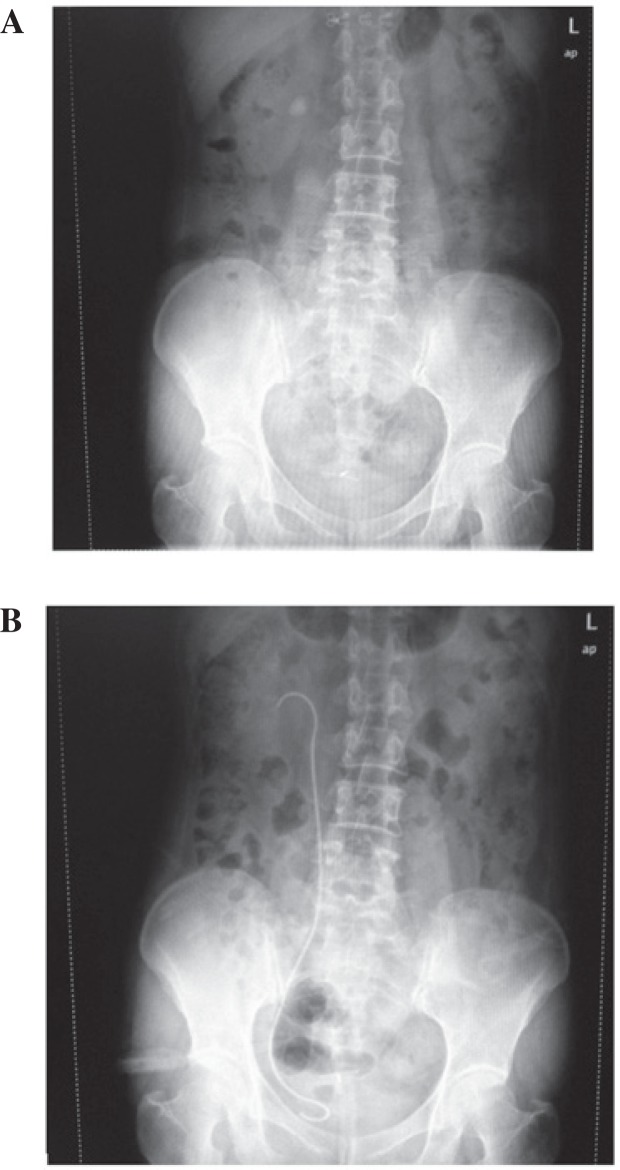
(A) Preoperative kidney, ureter and bladder (KUB) radiograph of Case 2, showing a stone at the right pelvis (diameter 1.8 cm). (B) KUB radiograph of Case 2 at day 1 postoperatively, indicating that the stone at the right pelvis was completely removed.
